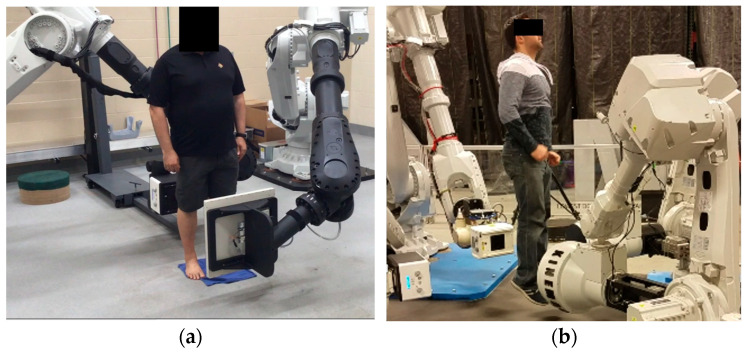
The “one-device–multiple-modalities” principle of the robotic scanner. (a) Patient’s 3D CT scan during standing and load bearing; (b) patient being scanned during load bearing and high-speed movement (jumping) with a set of multiple and different emitters/detectors.
